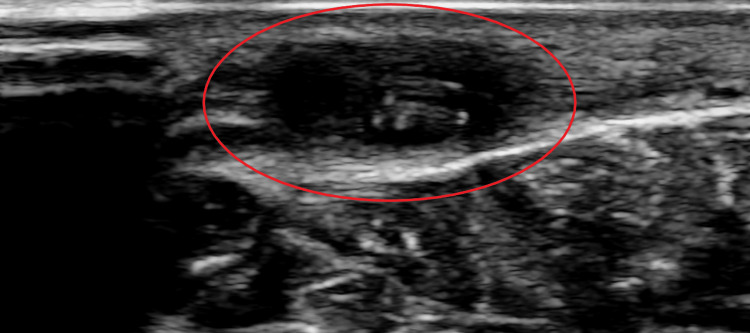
Ultrasonography Ultrasonography showing a subcutaneous hypoechoic lesion (red circle). Internal thin worm-like structures with parallel echogenic borders and anechoic centers can be seen.
